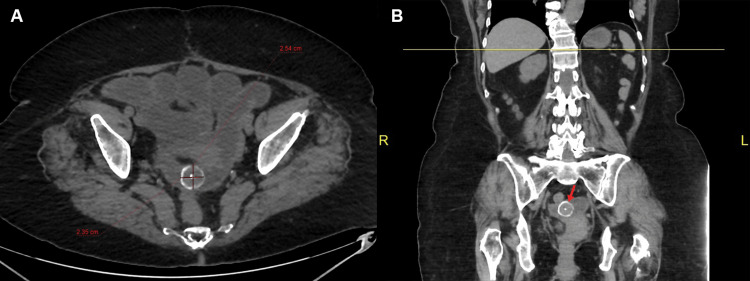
Non-contrast-enhanced computed tomography showing a 2.35 x 2.54 cm object inside intestinal loops (red arrow)
